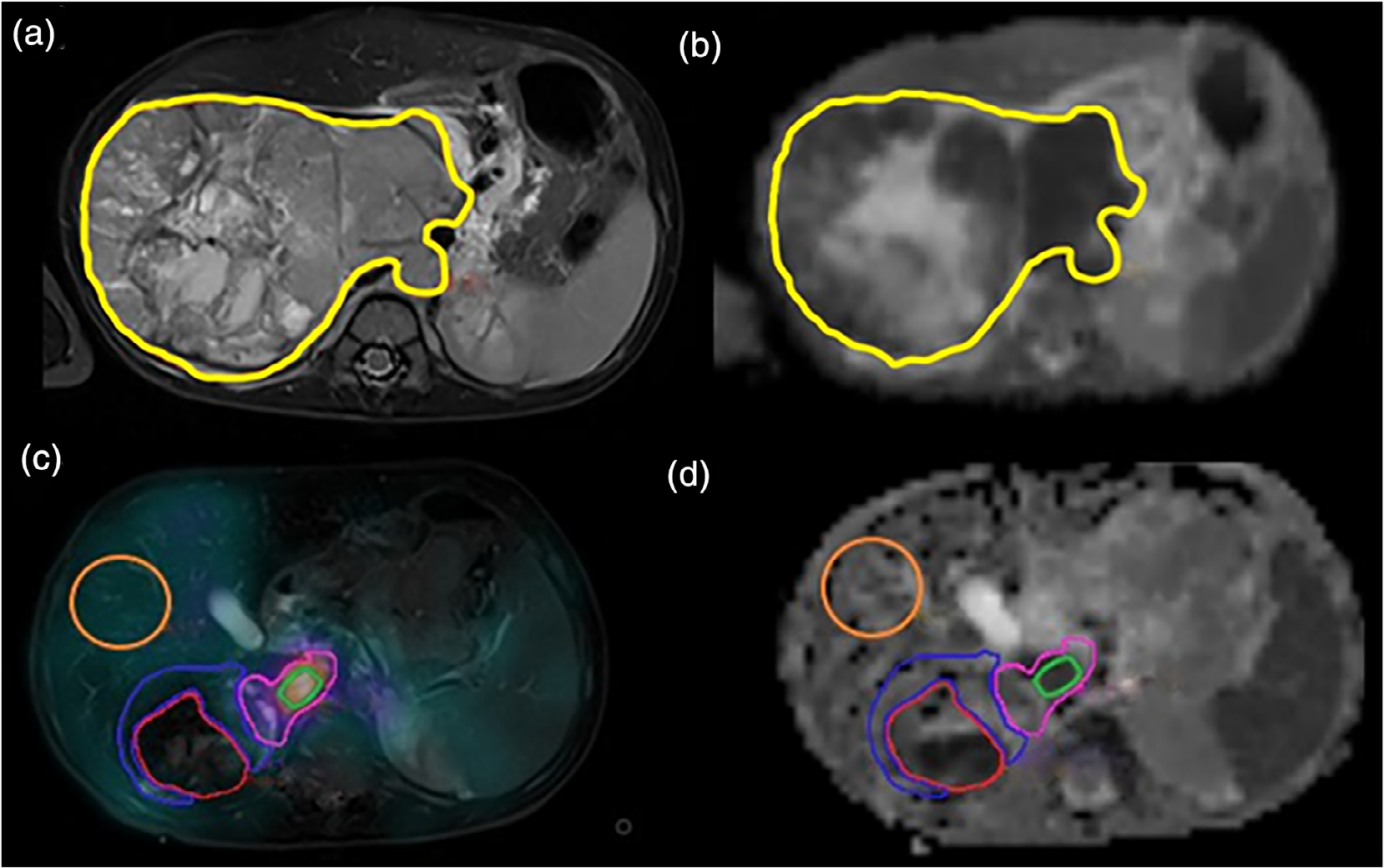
Representative images from the same patient (male, 20 months of age at diagnosis) before and after induction chemotherapy. Coregistered axial T_2_‐weighted image (a) and apparent diffusion coefficient (ADC) map (b) acquired prior to chemotherapy with the primary tumor ROI overlaid (yellow line). SPECT ^123^I‐mIBG image overlaid on T_2_‐weighted image (c) and ADC map (d) acquired postchemotherapy with the liver ROI (orange) used for normalizations and tumor subregion ROIs shown: high ^123^I‐mIBG avidity (green), moderate avidity (pink), low avidity (blue), and no avidity (red). In this example the “high avidity” ROI corresponded with the most avid part of the entire tumor volume.
